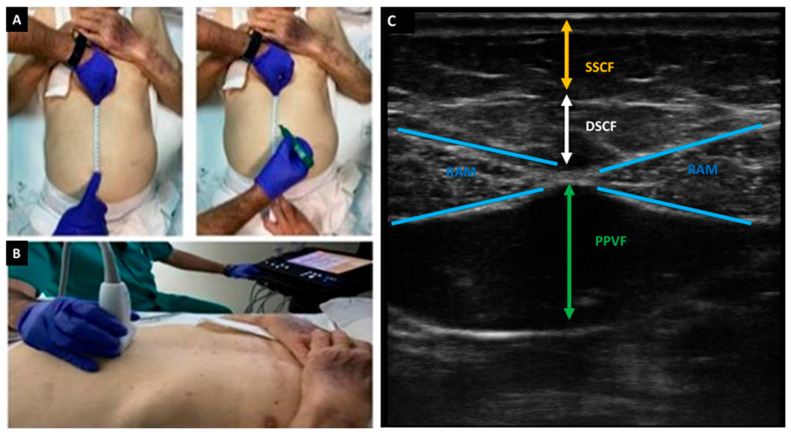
Systematic study (A,B) and ultrasound anatomy of preperitoneal visceral fat (PPVF) (C). SSCF: superficial subcutaneous fat; DSCF: deep subcutaneous fat; RAM: rectus abdominis muscle.
